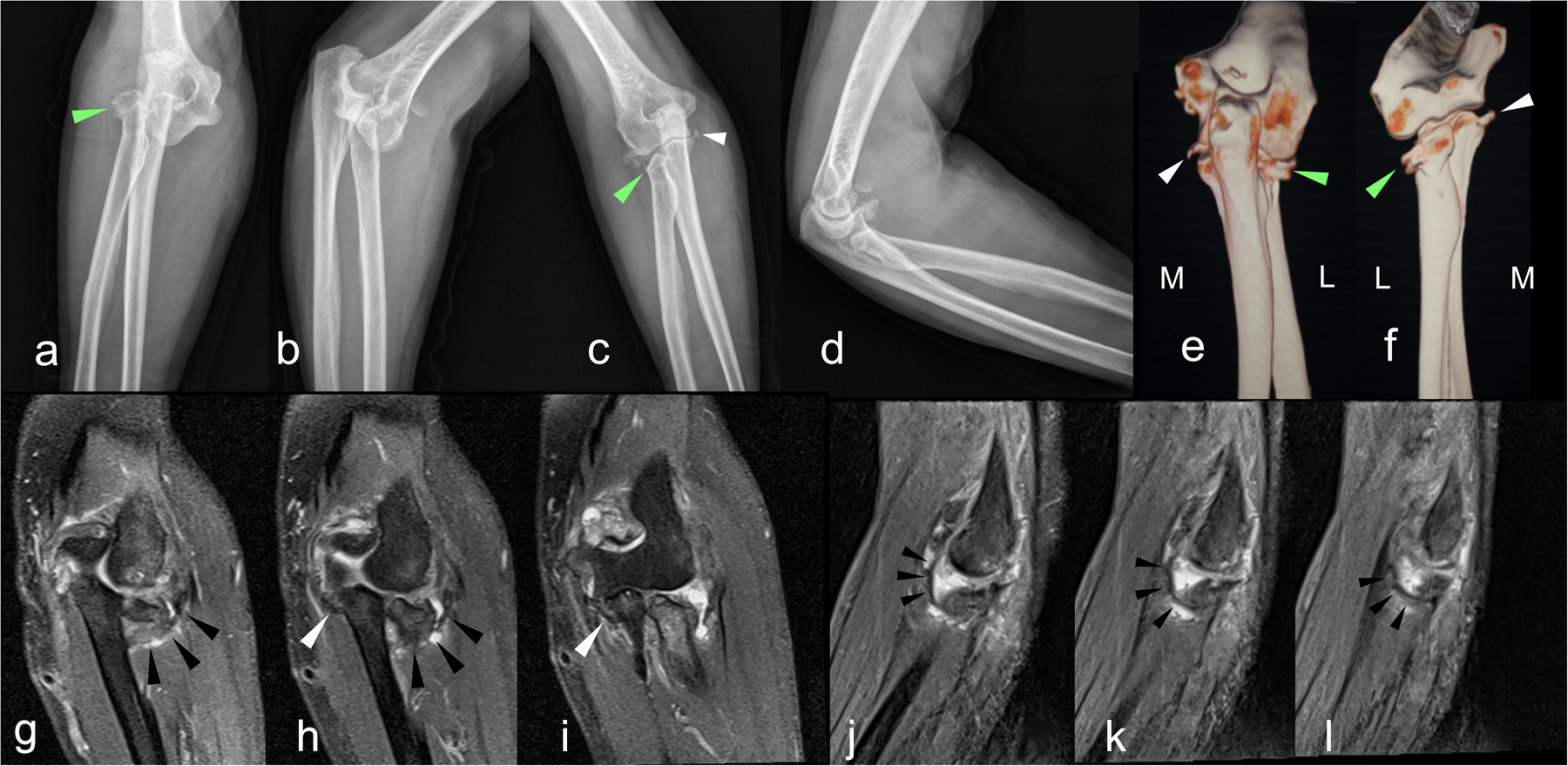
A 70-year-old woman fell on her extended left arm (case 1). Radiographs demonstrated a posterolateral dislocation (a,b); after the reduction (c,d), the laterally angulated radial head impaction and coronoid fragment can be found. (e,f) The back and front view of the forearm bones on 3D CT show the laterally impacted radial head (green triangles) and the avulsed coronoid fragment (white triangles), located at the anteromedial tip of the coronoid process. Continuous coronal (g–i) and sagittal (j–l) MRI slices confirmed the continuity of the LUCL (black triangles) and the MCL-related coronoid avulsion. In sagittal MRI views, distinguishing between the LUCL and annular ligament was sometimes difficult. Bone contusion of the radial head was more extensive than that of the coronoid, which was limited to the area surrounding the avulsion. These findings suggest a valgus mechanism. L, lateral; M, medial.
